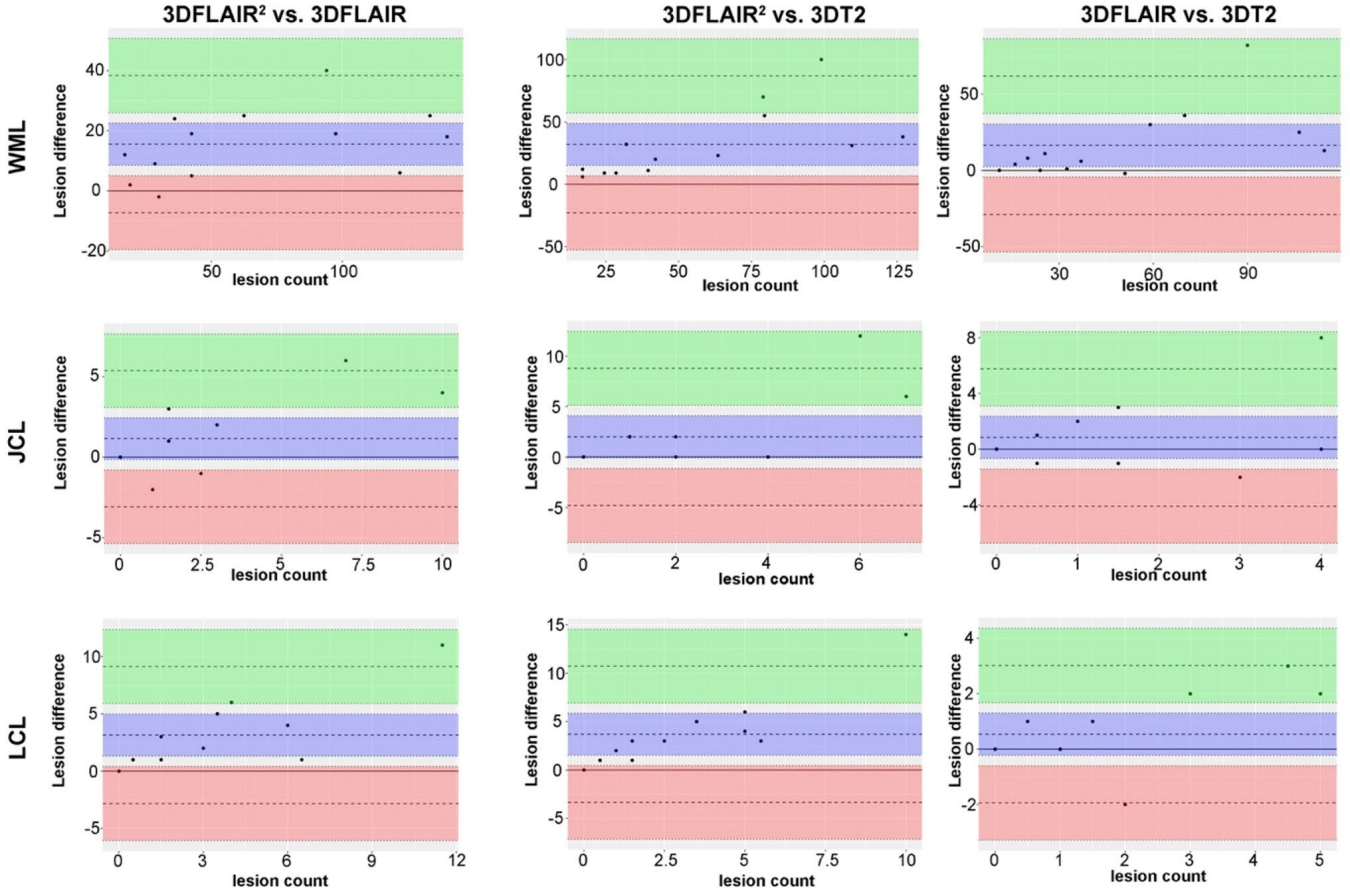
Difference in the numbers of assessed lesions. Bland–Altman plots comparing lesion counts derived from 3D-FLAIR^2^, 3D-FLAIR and 3D-T2 in each patient and each location. For white matter lesion (WML), juxtacortical lesion (JCL) and leucocortical lesion (LCL), the difference between lesion counts derived from the different sequences (3D-FLAIR^2^, 3D-FLAIR and T2), is plotted relative to their mean for each patient with black dots. The black dashed line provides the mean difference with the corresponding 95% CI in blue. The limits of agreement are provided with their 95% CI, the upper bound in green, the lower bound in red
